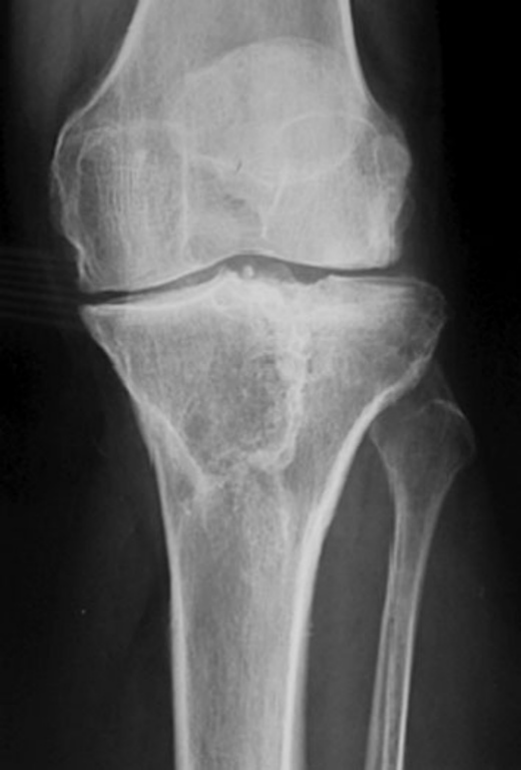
Schatzker type VI fracture, 1 year after X-rays (AP)
